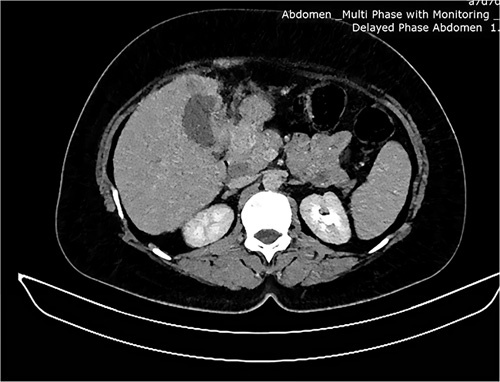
A computed tomography image showing enlarged liver and gallbladder mass.
